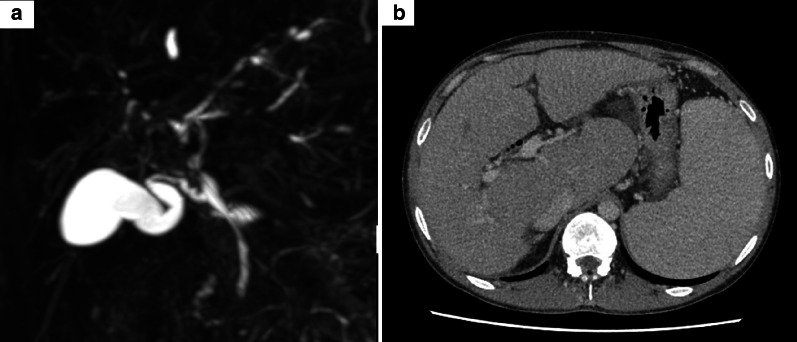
The imaging findings of primary sclerosing cholangitis (PSC) are shown. a Magnetic resonance cholangiopancreatography revealed typical features of PSC with irregular narrowing of bile ducts, stenoses, and focal dilatation of bile ducts. b Computed tomography revealed a cirrhotic liver with an enlarged caudate lobe and splenomegaly
